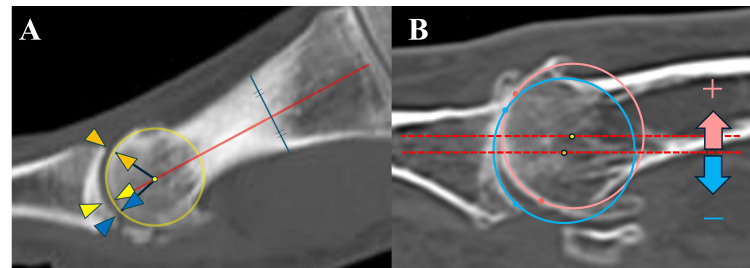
Measurement parameters on weight-bearing CT for hallux rigidus evaluation. (A) MTP joint space width: Measured at three points: the dorsal edge of the proximal phalanx articular surface, the extension line of the first metatarsal shaft axis, and the plantar edge. (B) Deviation of the center of rotation: A best-fit circle was drawn along the articular surfaces of the proximal phalanx and metatarsal head to determine each center of rotation. A negative value was assigned when the center of the proximal phalanx was plantar to that of the metatarsal head.
